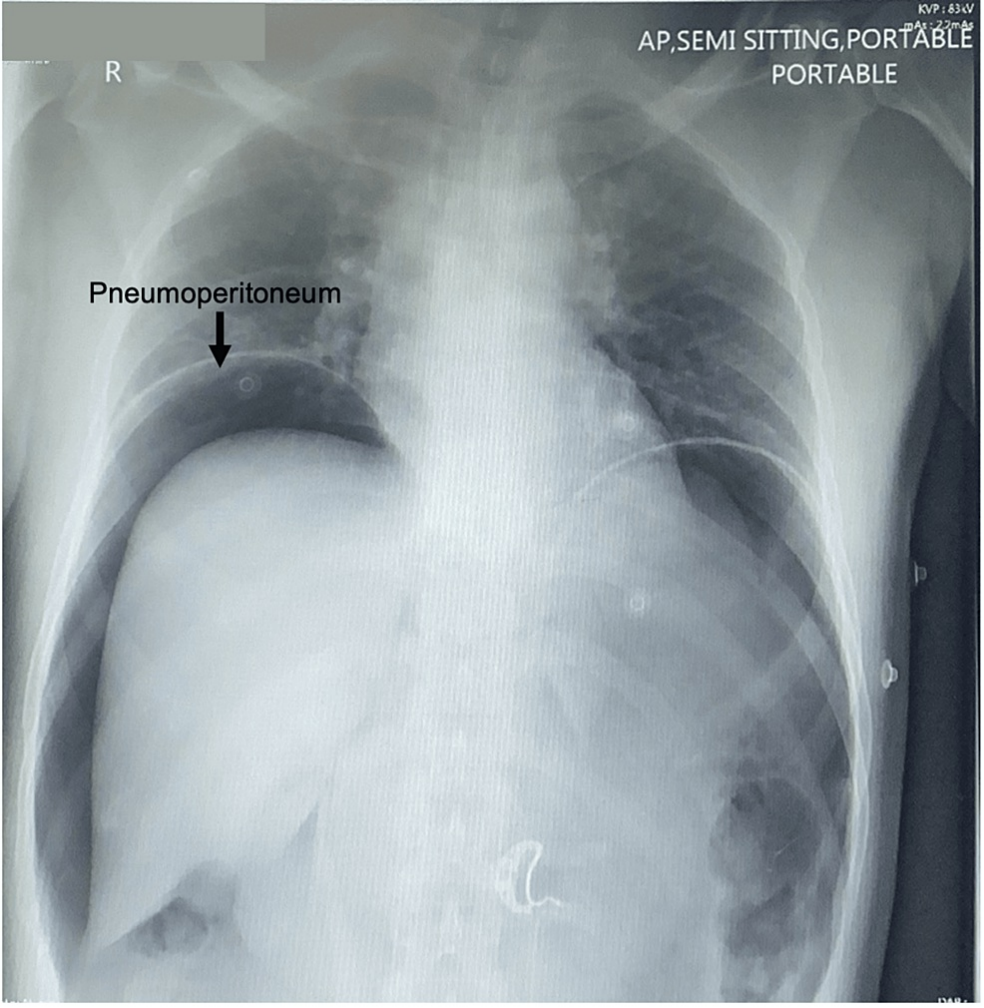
Semi-sitting portable chest X-ray showing bilateral marked pneumoperitoneum
